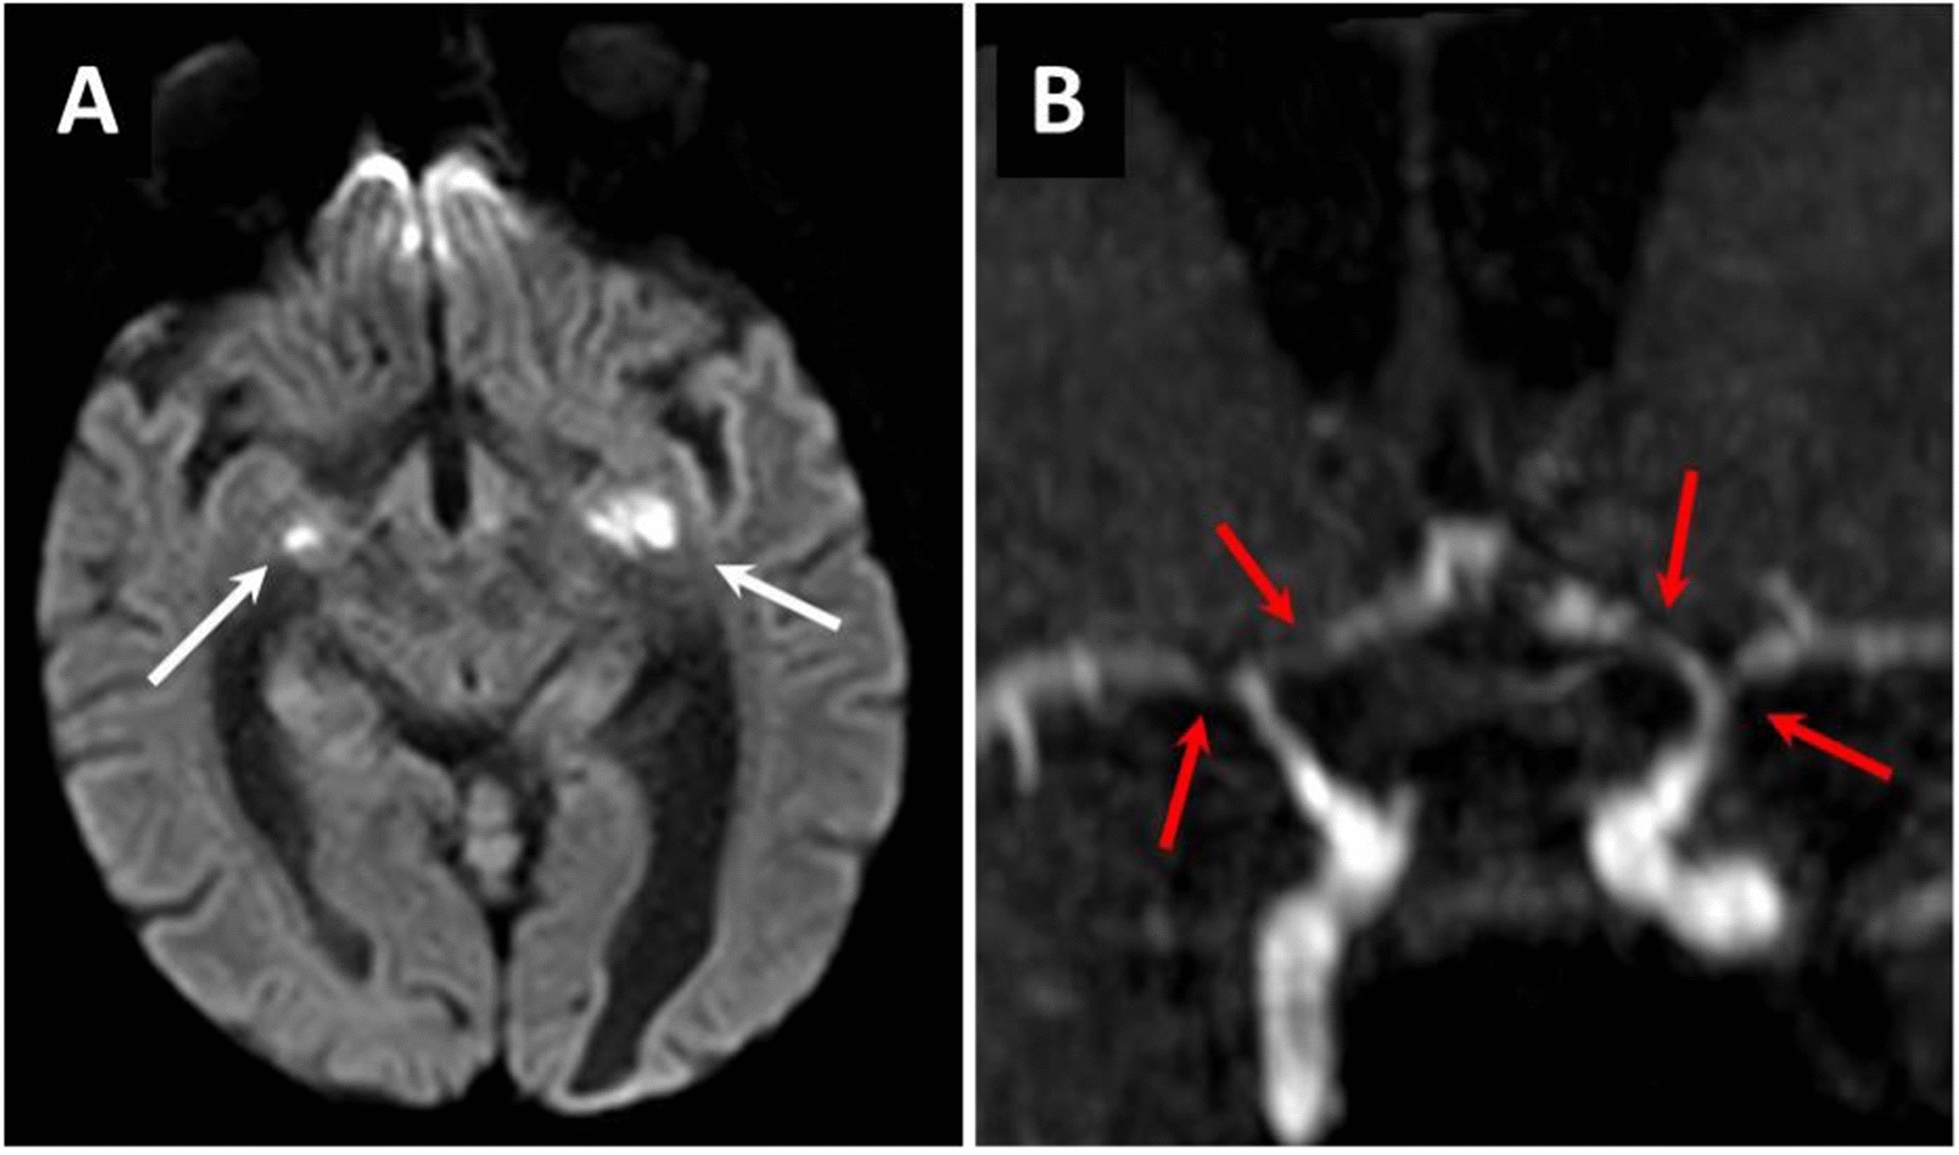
Imaging characteristics of VZV encephalitis and vasculitis from brain MRI. A 18-year-old man was admitted to the ICU for fever, headaches and altered consciousness. He underwent a second kidney transplantation 2 years ago. The onset of neurologic symptoms started 2 days before ICU admission and invasive mechanical ventilation was implemented at day 2 for coma. The lumbar puncture revealed acute lymphocytic meningitis and the PCR for VZV was positive. Brain MRI showed multiple recent ischemic lesions of the brain parenchyma (A: white arrows) and vasculitis with multiple stenosis of the circle of Willis arteries (B: red arrows). He was treated with intravenous acyclovir 10 mg/kg/8 h and steroids (methylprednisolone 1 mg/kg). He recovered gradually and was extubated after 4 days of invasive mechanical ventilation. The patient was ultimately discharged alive 8 days after ICU admission
